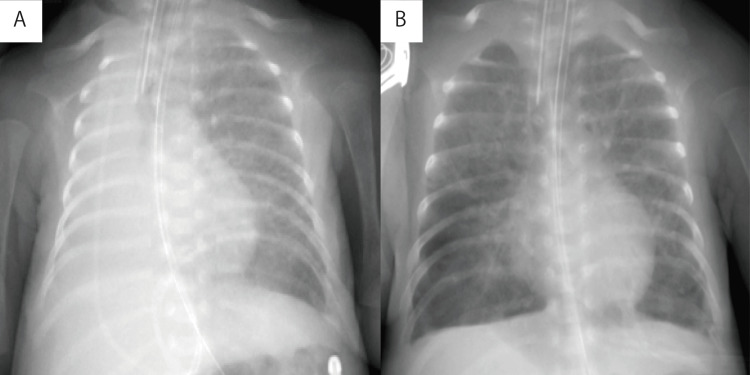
Chest radiographs Right pulmonary atelectasis (A) followed by the condition after surfactant lavage (B) was indicated
